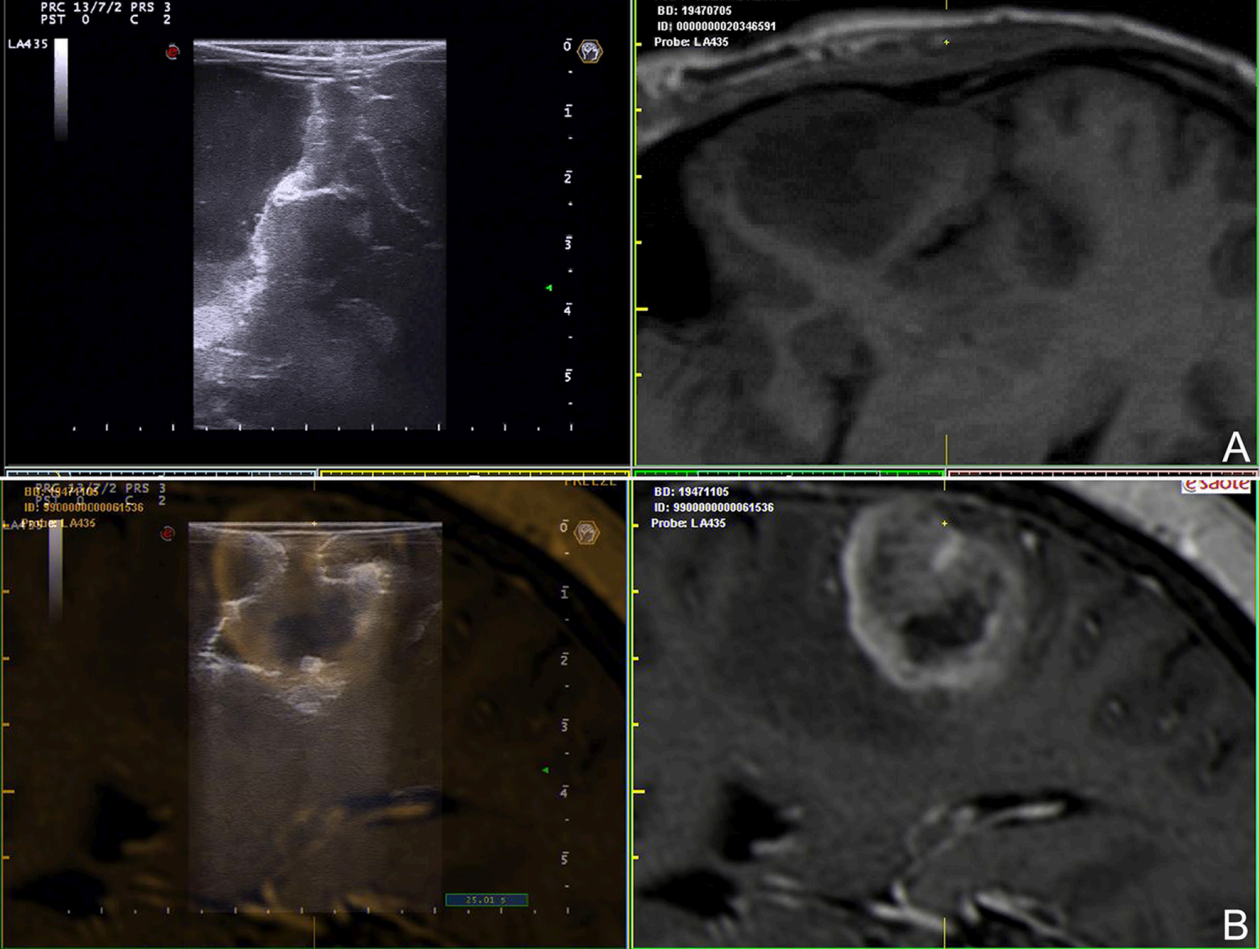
Navigated intra-operative B-mode US in the final stages of surgery. (A) Left temporal anaplastic astrocytoma. (B) Right fronto-temporal glioblastoma. The comparison between ioUS and corresponding pre-operative MRI aid in identifying residual tumor and understanding ioUS semiotics: (A) side-by-side view, (B) superimposition.
